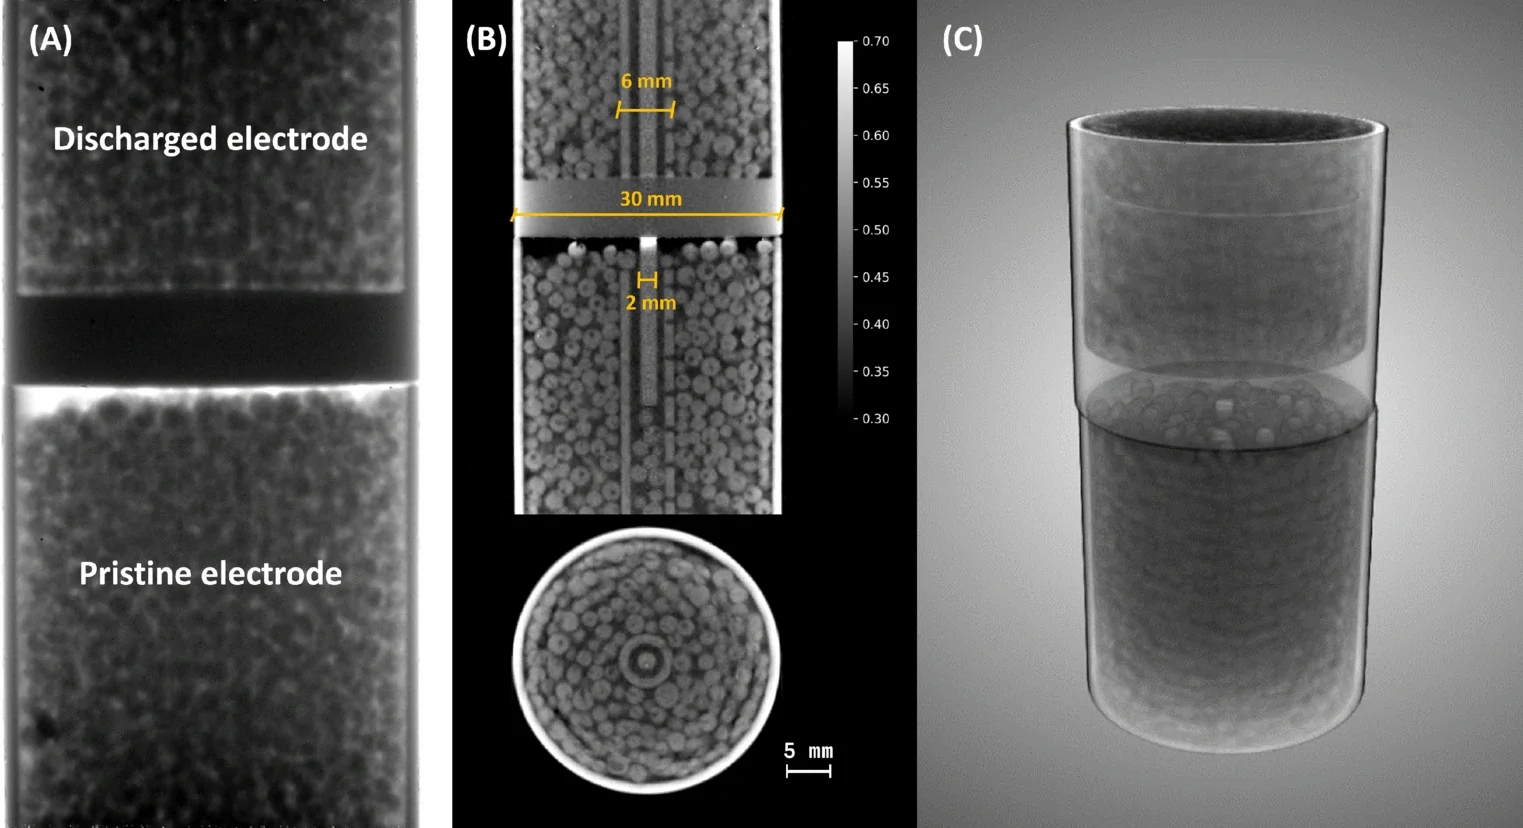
(A) Example of a single, post-processed X-ray lateral radiography; (B) Sagittal (top) and axial (bottom) slices of the 3D tomographic reconstruction. (C) 3D volume rendering of the tomographic reconstruction.
